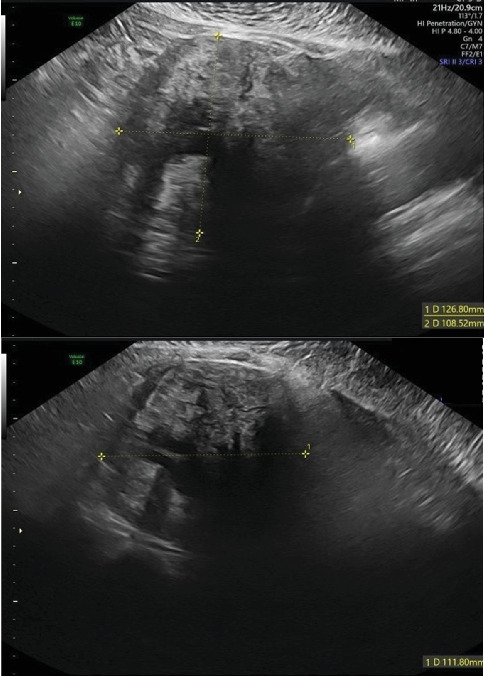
Transvaginal ultrasound, performed in the ultrasound unit of the gynecology department before the surgery, showed a pelvic heterogeneous solid mass, measuring 127 × 109 × 112 mm, with areas of cystic degeneration and without any apparent connection to the uterus.
